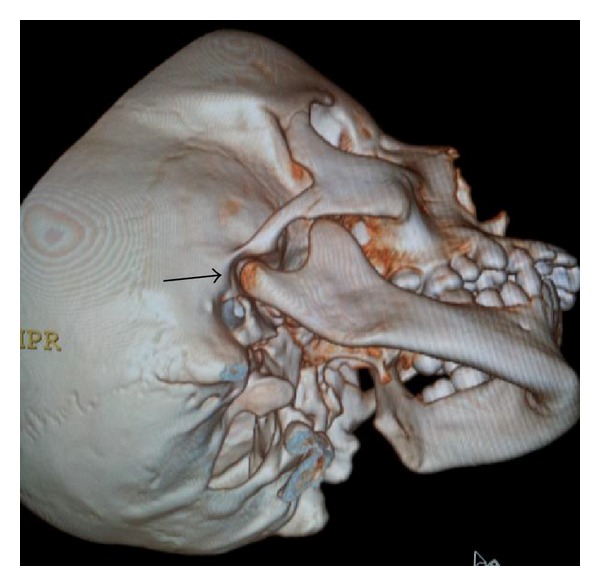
3D CT scan of normal side (right) with normal condyle and glenoid fossa.
